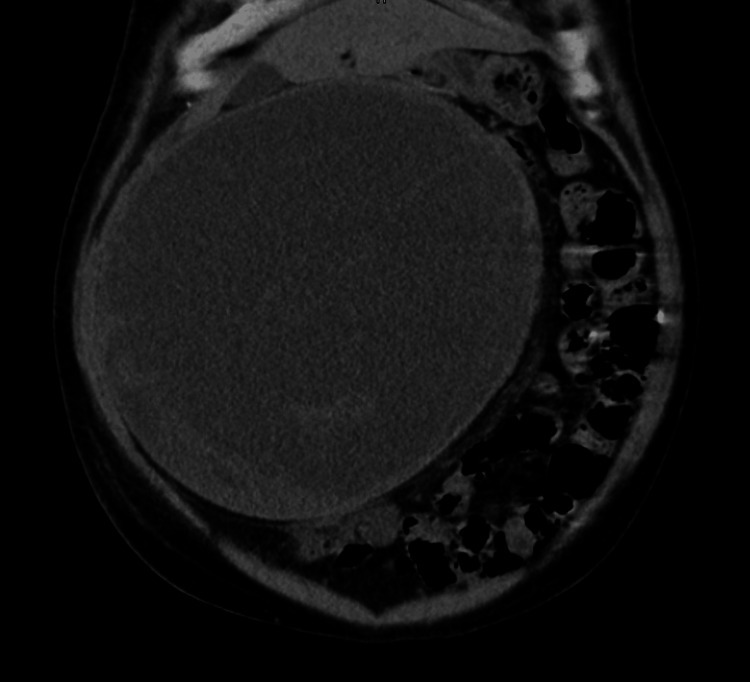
Coronal CT Image of the abdomen Coronal CT image of the abdomen depicting a large cystic lesion originating from the inferior border of the liver. The cyst features thin enhancing septations without any solid components or calcifications. There is no evidence of intrahepatic biliary dilation, and no other hepatic lesions are present. The mass exerts a significant mass effect on the adjacent bowel loops, indicating potential displacement.
